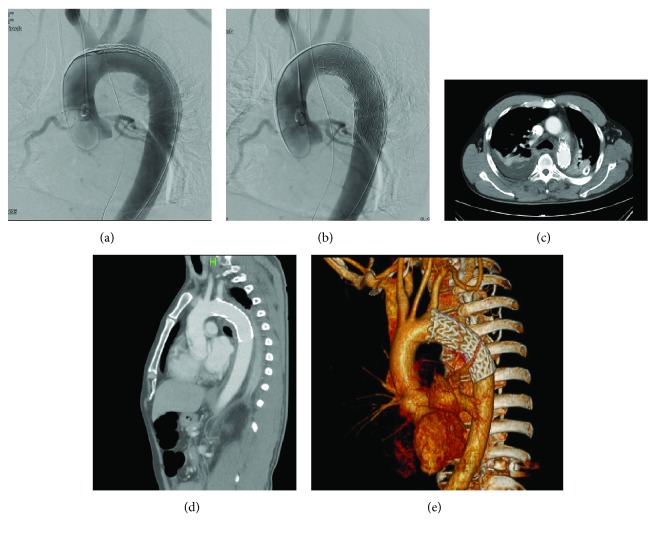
Case 1. Intraoperative aortography: (a) before deploying the stent graft and (b) a pseudoaneurysm was observed but it disappeared after the stent graft was deployed. Postoperative computed tomography: (c) axial image, (d) sagittal image, and (e) 3-dimensional image. The pseudoaneurysm had disappeared.
